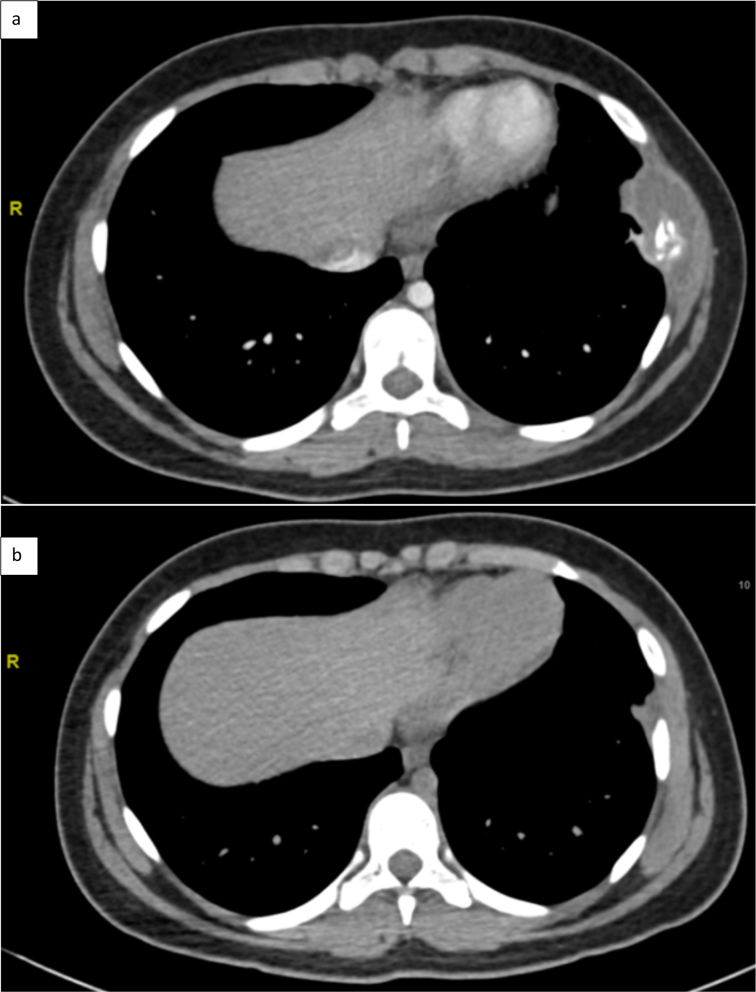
Thoracic CT scan (a) at diagnosis (b) end of treatment.
